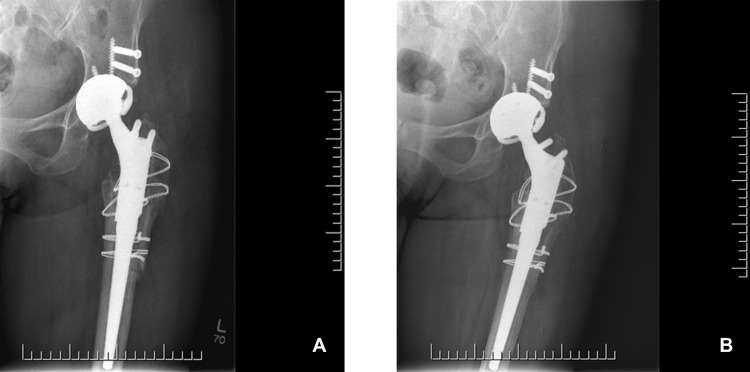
Follow-up radiographs Post-operative radiographs at 7 months (A) and 11 months (B) showed hardware in stable position.
